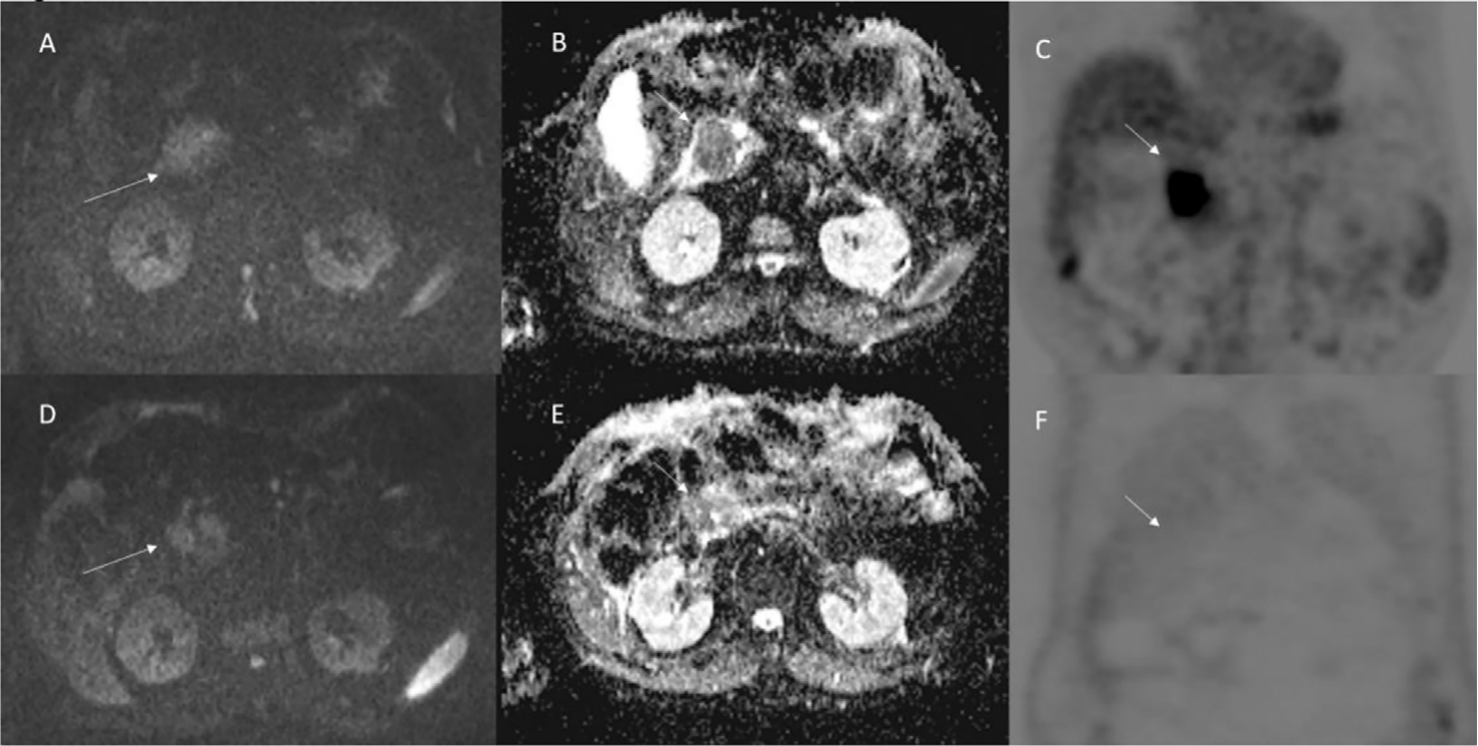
Patient 3 with head pancreatic cancer (the same of Figure 4). Functional (DW-MRI and PET) assessment post-ECT treatment. In (A) (pre treatment) and (D) (post treatment) (b 800 s/mm^2^) the lesion (arrow) shows restricted signal so as in ADC map (B: pre treatment; and E: post treatment) the lesion shows hypointense signal (arrow). This qualitative analysis is indicative of non-responder lesion. The PET (C: pre treatment; and F: post treatment) assessment shows (arrow) a responder lesion.
